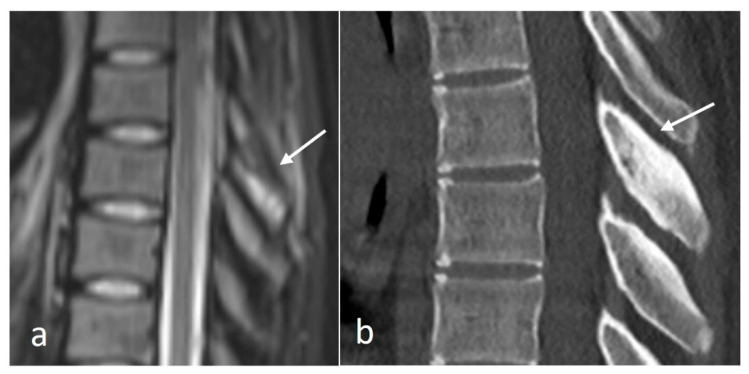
Sagittal STIR (a) and CT (b) images demonstrating a thoracic spinous process osteoid osteoma (white arrows). Note the presence of reactive marrow oedema on the STIR sequence. The sclerotic reactive bone is well delineated on CT, although the lucent nidus may not always be present as in this case.
